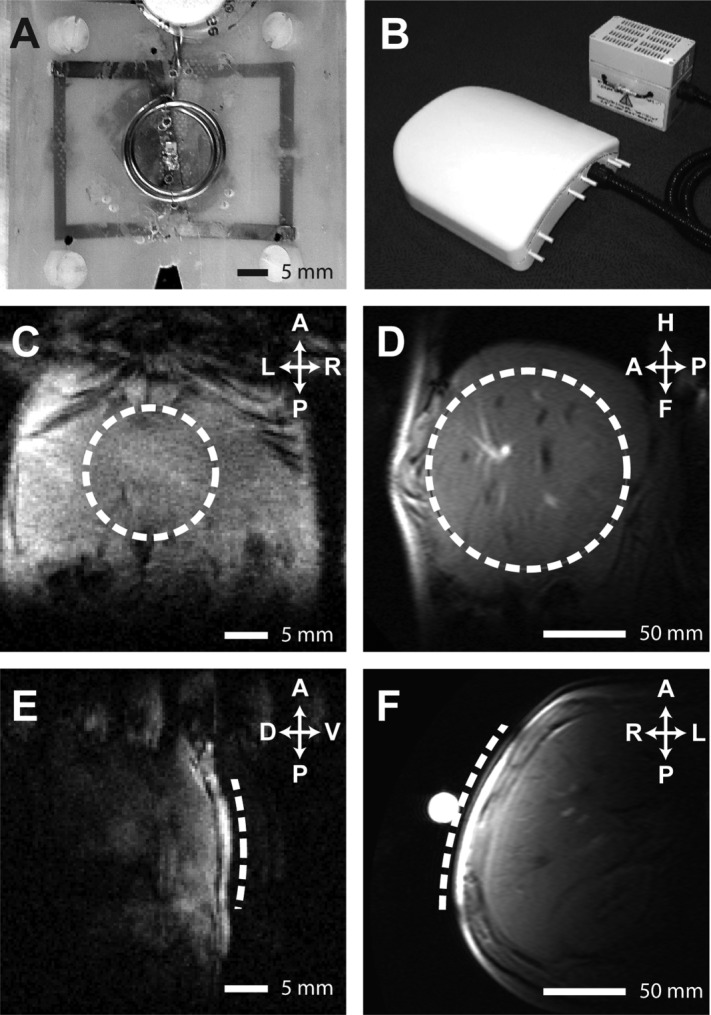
^13^C/^1^H RF coils, and MR images showing coil positioning. (A and B) ^13^C/^1^H RF coils employed for preclinical and human studies, respectively. (C-F) Representative hepatic ^1^H images showing location of ^13^C surface coils (dashed line) for preclinical (C and E) and human (D and F) studies.
